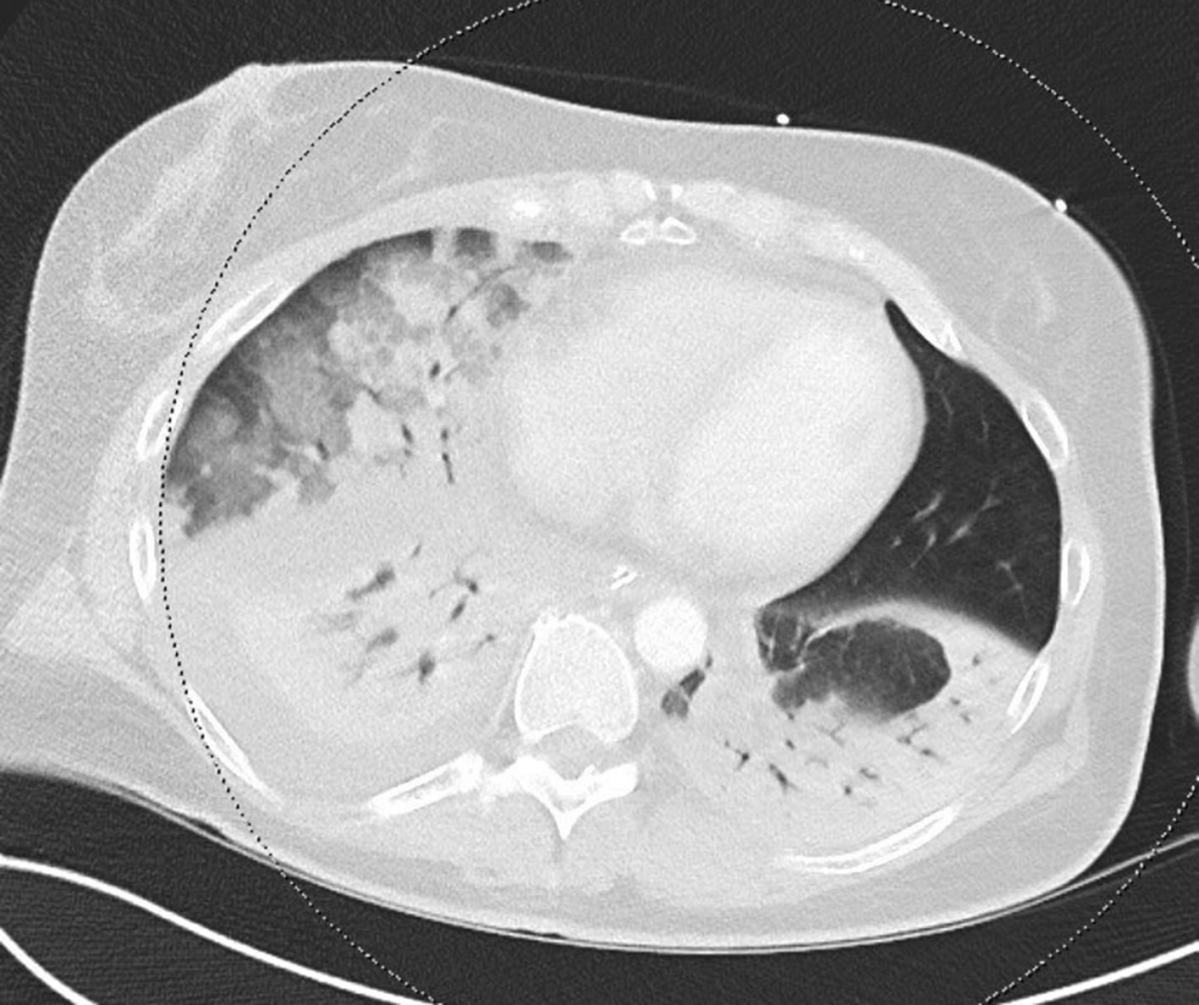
Thoracic CT scan showing bilateral pneumonic infiltrations and right-side pleural effusion.
